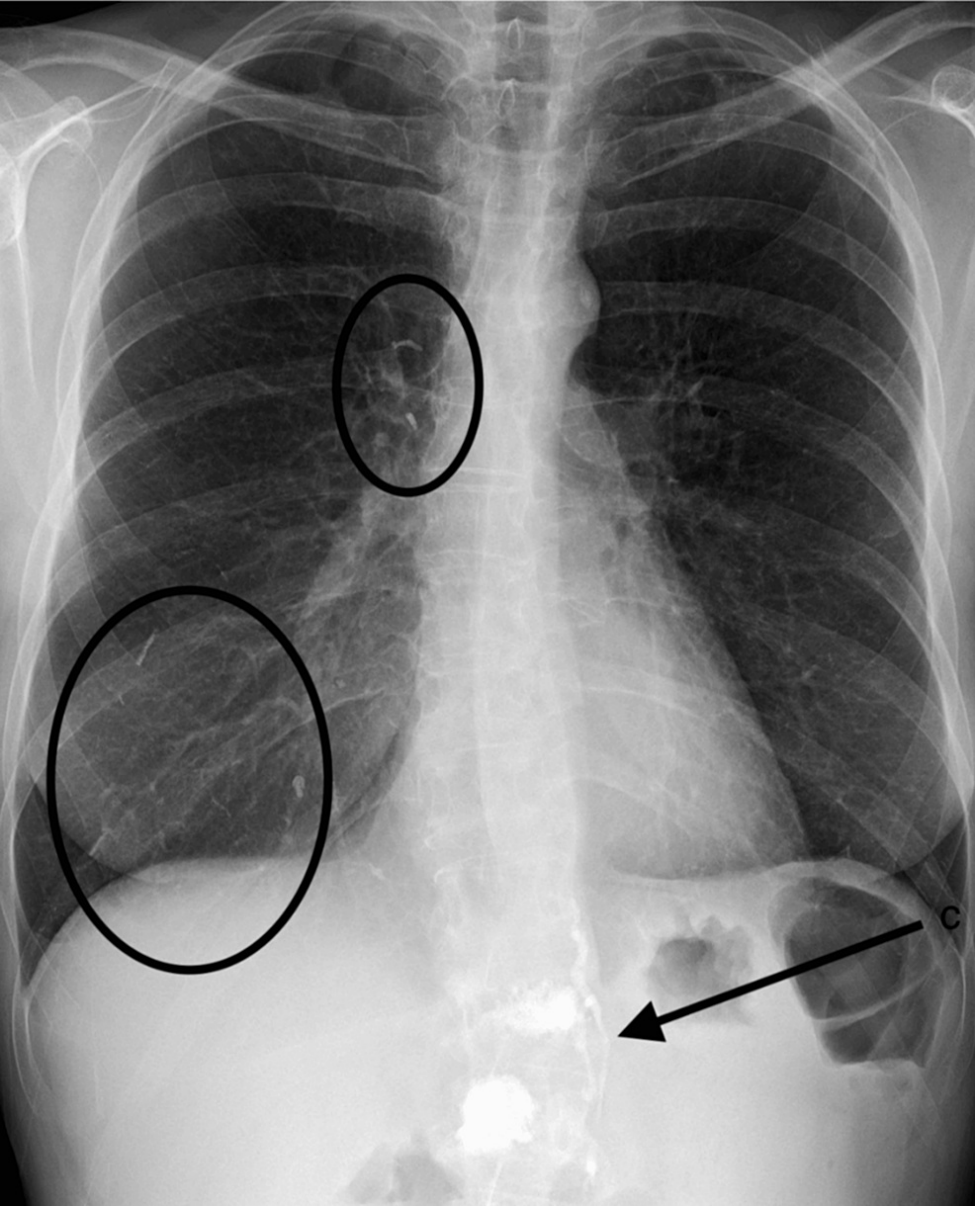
Chest X-ray. The arrow shows polymethylmethacrylate (PMMA) extravasation in the paravertebral veins. The circles show embolization of the lungs.
